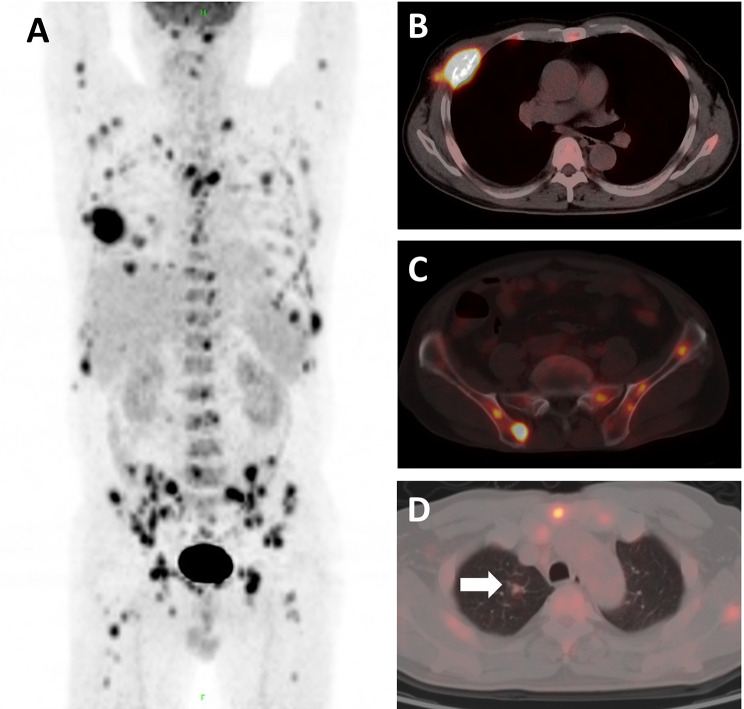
A 47-year-old man (No.2) presented with right calf and chest pain, fever, and sweating for one month. Laboratory examination revealed significant increases in leukocyte count (19 × 10^9^/L), erythrocyte sedimentation rate (77 mm/hour), and C-reactive protein level (87 mg/L). ^18^F-FDG PET/CT showed diffuse bony destruction with increased high FDG uptake (SUV_max_ = 14.8) and mild-uptake small nodule in the upper lobe of the right lung (white arrows, SUV_max_ = 2.0). Finally, *M. intracellulare* was confirmed by sputum culture of a rib lesion after removal in orthopedics
